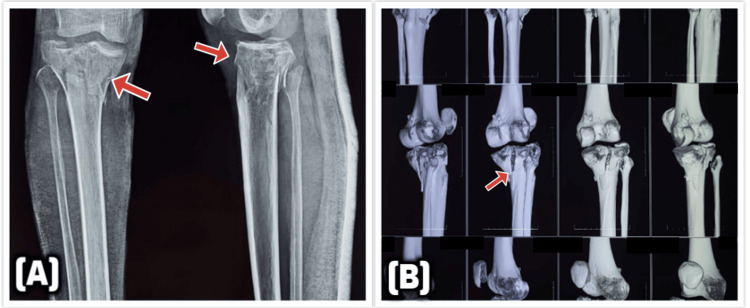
Preoperative radiographic and CT evaluation of a bicondylar tibial plateau fracture (A) AP and lateral radiographs showing a bicondylar tibial plateau fracture with depression and displacement of both medial and lateral condyles (red arrows). (B) 3D-CT reconstructions further delineate the fracture morphology, comminution, and joint surface involvement, aiding in surgical planning (red arrow indicates fracture line). CT: computed tomography; AP: anteroposterior
